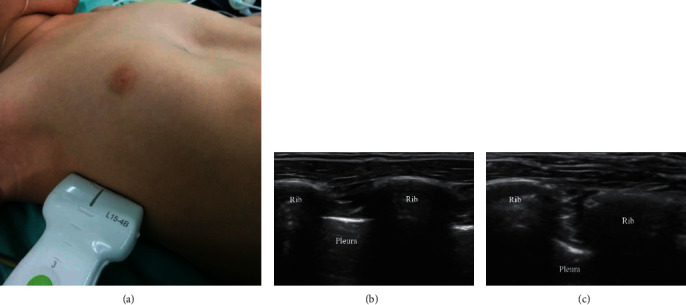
Ultrasound image of intercostal nerve blocks. (a) Placement of the ultrasound-probe for the performance of intercostal nerve block. (b) The hyperechoic ribs and the pleura. (c) After the administration of the local anesthetic, the bolus increased, and expansion of the potential space is visible.
